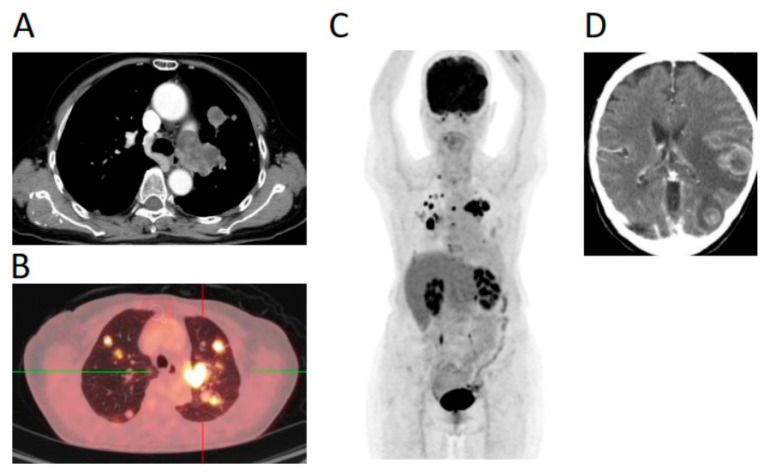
CT images (A,D) and PET/CT scans (B,C). (A,B) Primary pulmonary artery sarcoma (PPAS) with lung metastases by CT scan (A) and the corresponding PET/CT scan (B). (C) A whole-body PET scan showed PPAS with multiple metastases to the lung, bone, and brain. (D) Brain metastases on CT scan.
